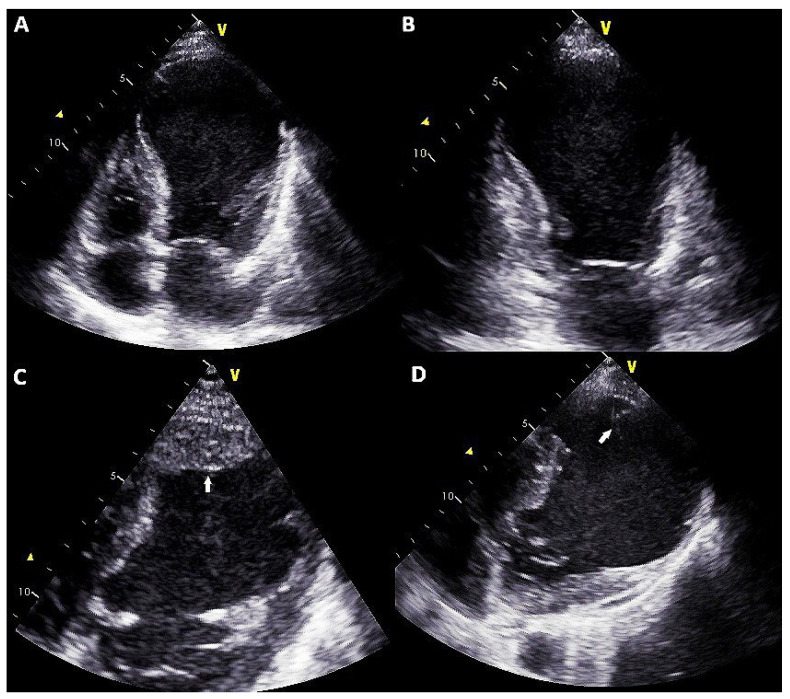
Transthoracic echocardiography four-chamber (A) and two-chamber (B) views showing apical aneurysm of the left ventricle. Focused off-axis view of the apical region showing thrombotic stratification (C) evolved into pedunculated and mobile thrombotic material (D) after anticoagulant treatment.
